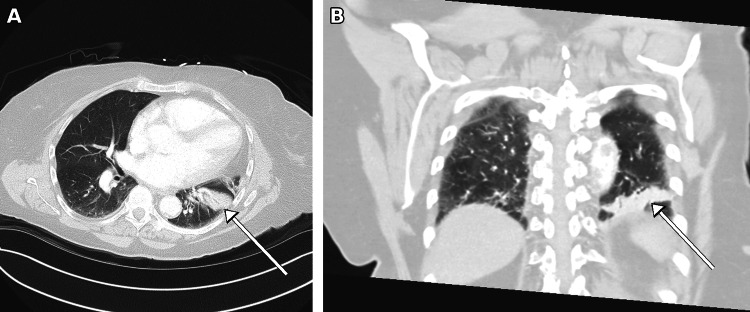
Contrast-enhanced computed tomography angiogram of the chest. (A) Axial image demonstrating patchy consolidation in the left lower lobe (arrow). (B) Coronal reformat confirming the basal location of the left lower lobe consolidation (arrow).
